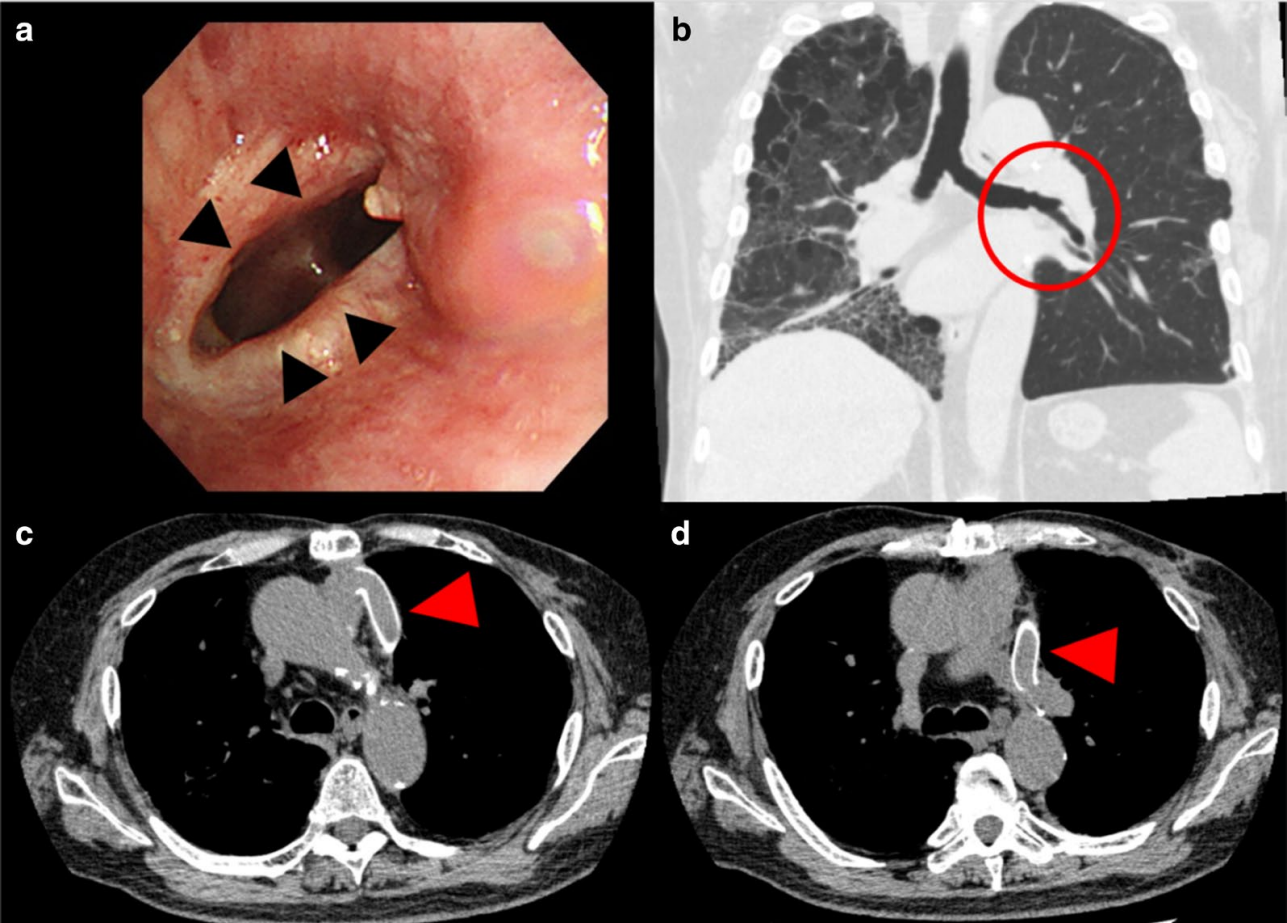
Postoperative bronchoscopic image and chest computed tomography scans 10 months after reconstructive surgery. a Anastomosis of the bronchus (black arrowheads). b Coronal section of a computed tomography scan showing the patent’s left main bronchus (red circle). c, d A vascular graft (red arrowhead) extending from the pulmonary artery trunk to the left main pulmonary artery
